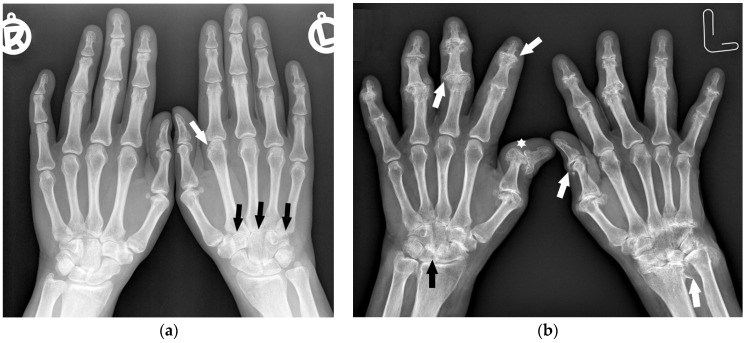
A 40-year-old female with early asymmetric seropositive rheumatoid arthritis (RA). (a) Posterior–anterior (PA) radiographs of bilateral hands show narrowing of the left radiocarpal joint (RCJ) and carpo-metatacarpal (CMC) joints (black arrows). Note the bone cyst in the left second metacarpal head and narrowing of the second metacarpophalangeal joint (MCP) (white arrow). (b) PA radiograph of the bilateral hands of the same patient, 13 years later, showed progression of the disease with increased juxta-articular osteoporosis. Note the erosive bone changes, advanced destructive changes, and joint narrowing in the RCJ and CMC joints. There are less advanced changes on the right side with joint space narrowing and bone cysts in the wrist (black arrow). Note proliferative osteoarthritic changes in left distal radioulnar joint (DRUJ) and bilateral first MCP and interphalangeal joints (white arrows). There is subluxation of the right thumb interphalangeal (IP) joint (star).
